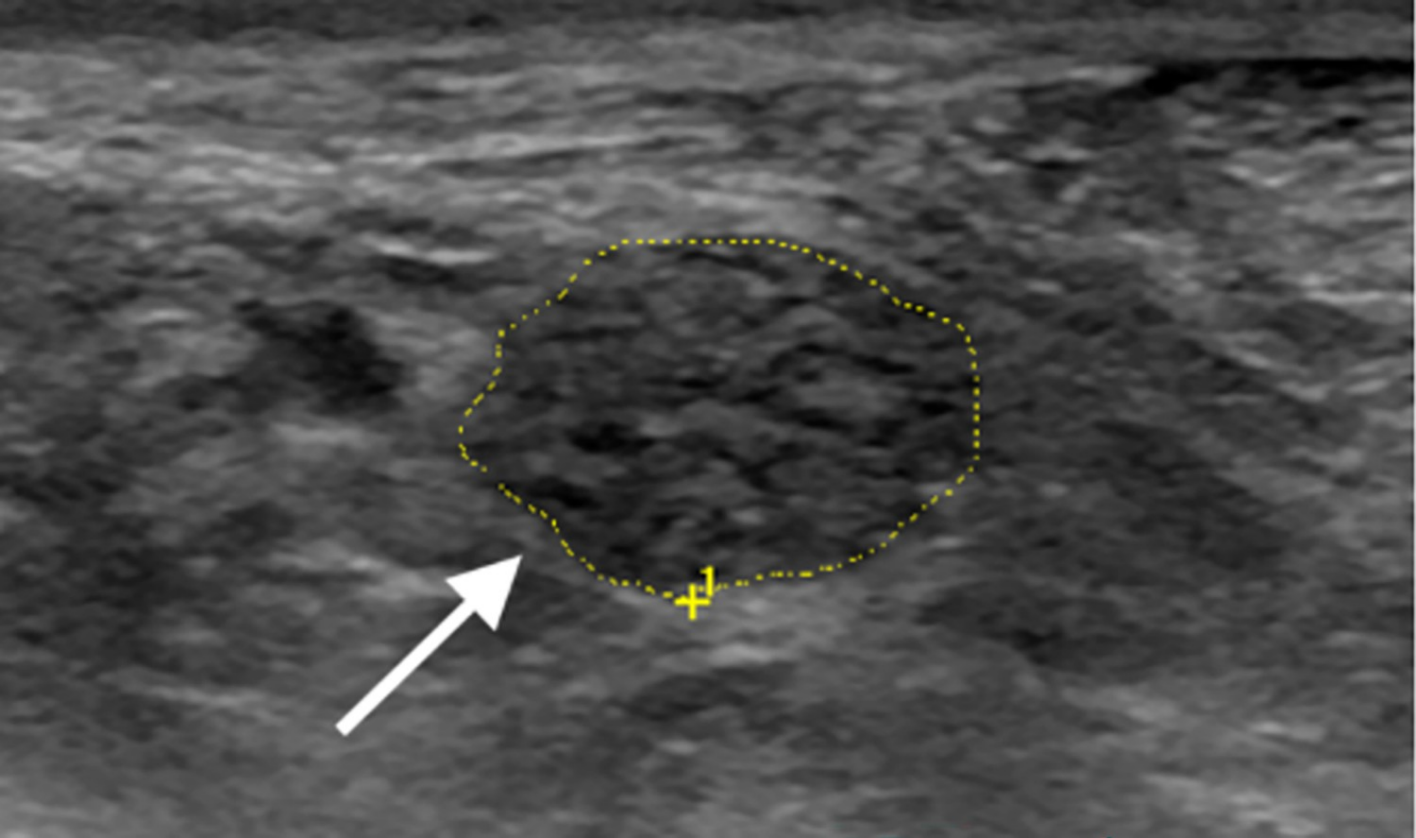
Ultrasound of the tibial nerve (transverse view) proximal to the tarsal tunnel in a leprosy patient, showing a thickened and hypoechoic nerve with loss of the fascicular pattern (white arrow).
